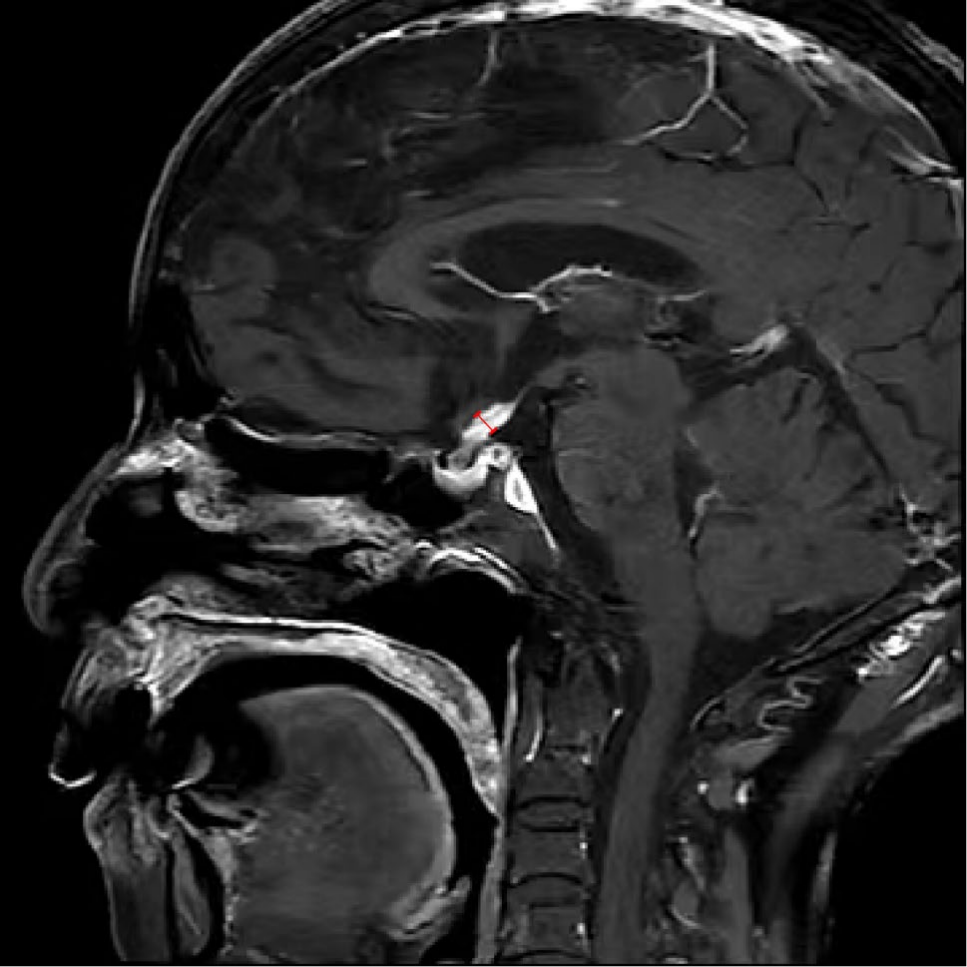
Magnetic resonance image of the pituitary gland. The T1 sagittal fat suppression sequence shows a thickened pituitary stalk with the widest point being 5.6 mm
